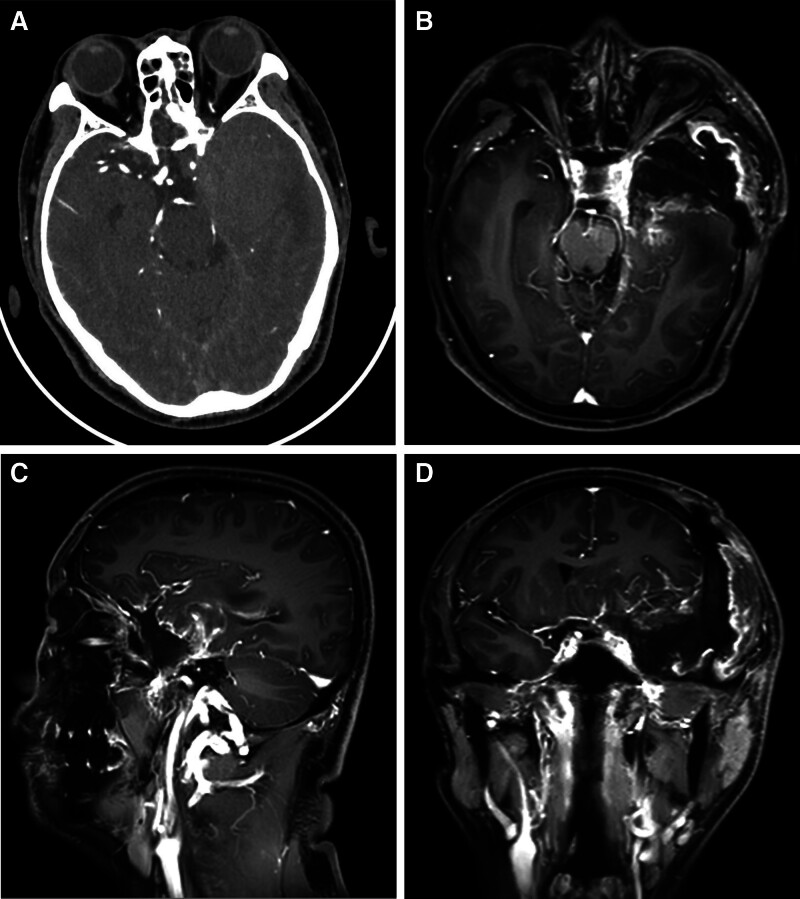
Postoperative CT scan and MRI show complete tumor resection. (A) CT scan, (B) axial MRI, (C) sagittal MRI, and (D) coronary MRI. CT = computed tomography, MRI = magnetic resonance imaging.
